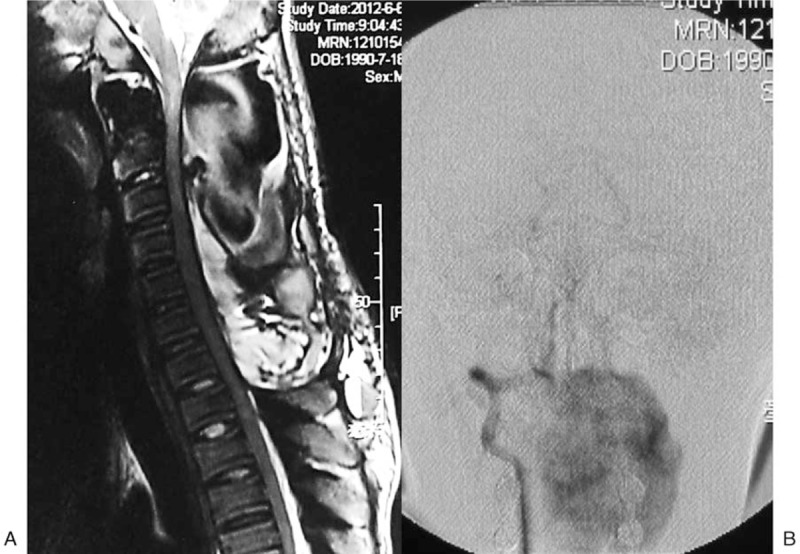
Cervical MRI and angiography on postoperative day 40. (A) MRI examination demonstrated a large epidural hematoma and extensive spinal cord compression. (B) The bleeding point was located at the right V3 segment in the VA groove of the atlas.
